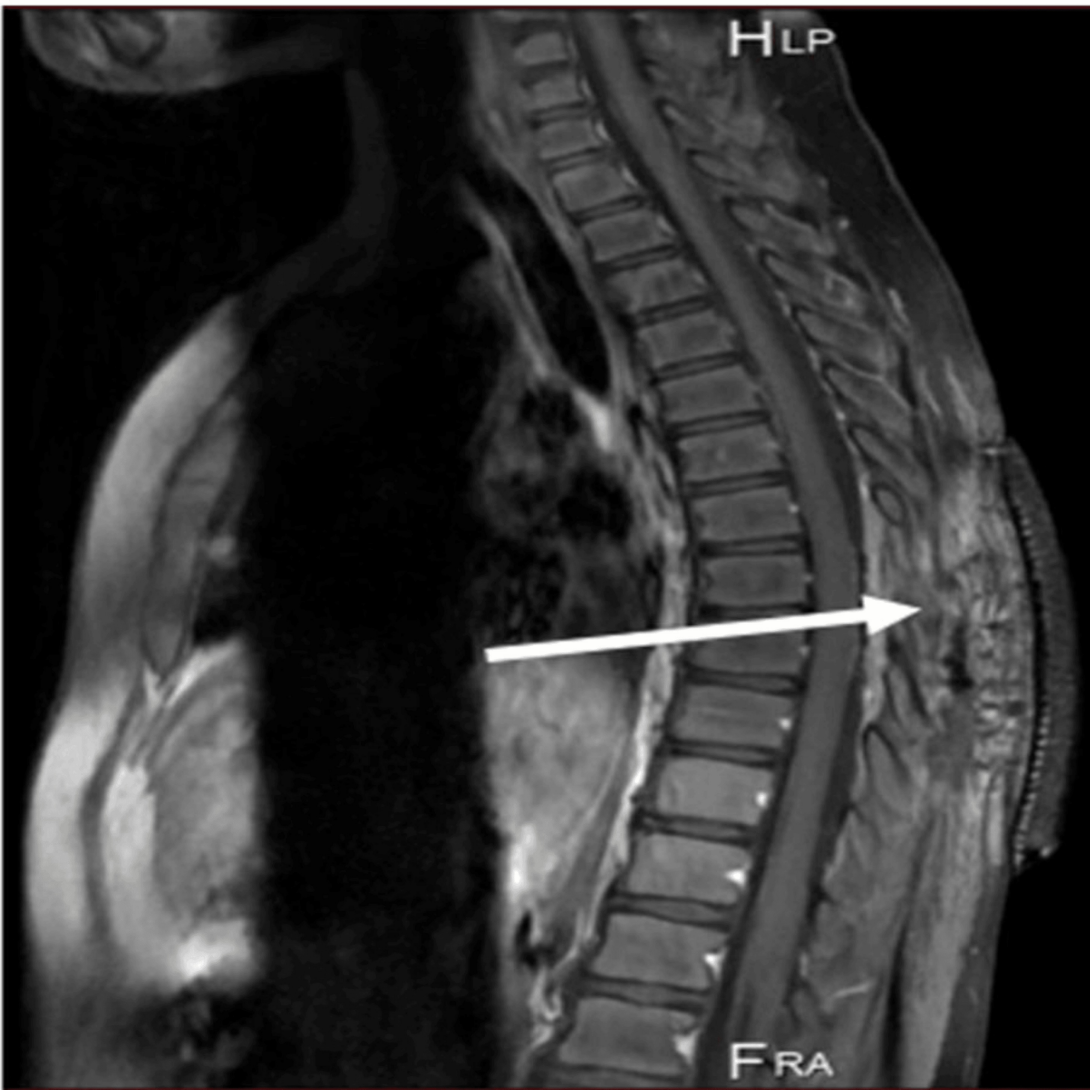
Spine MRI (T1 sagittal view) showing post-surgical excision of the tumor (arrow).
